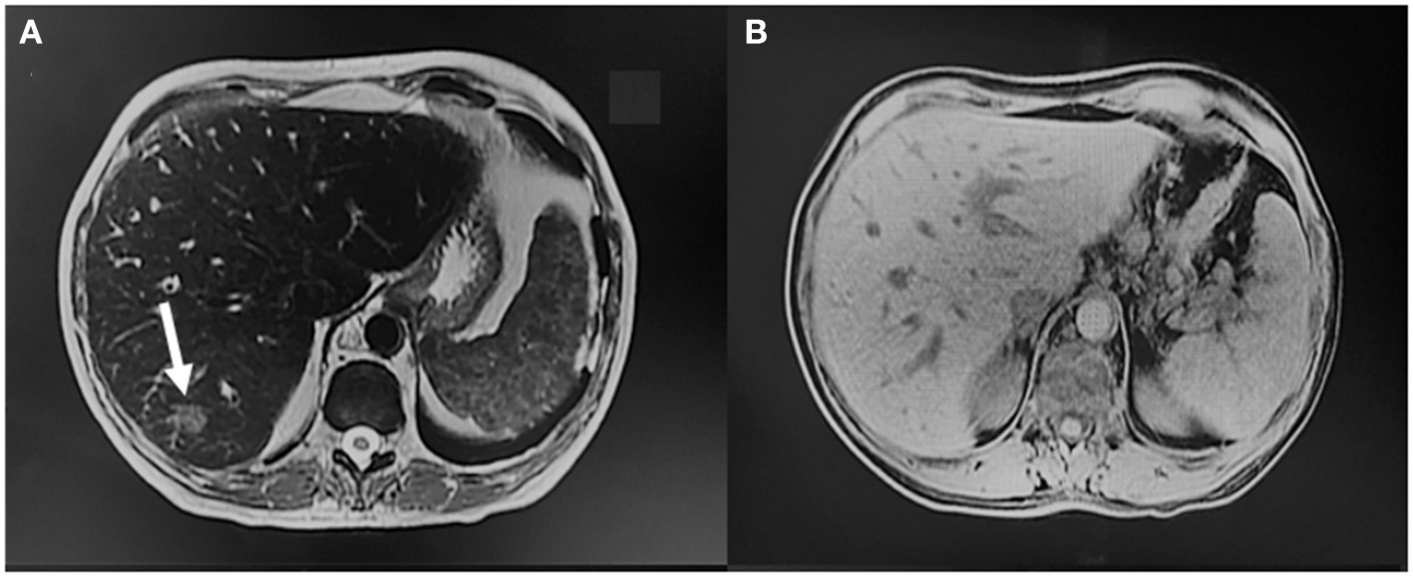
MRI findings during subsequent hospital admission. (A, B) Hepatomegaly and size reduction of the hepatic lesions previously described (arrow), with reduction of the multiple reactive abdominopelvic lymph nodes.
